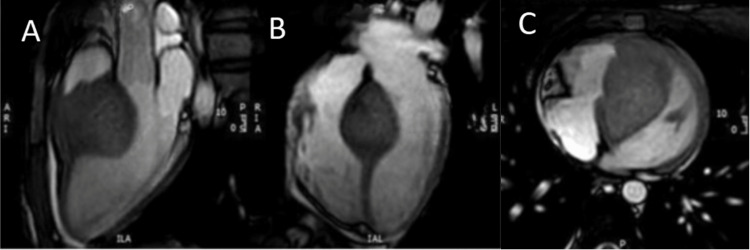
CMR imaging (A and B) SSFP of apical four-chamber and apical three-chamber images in sequence. (C) Mid axial SSFP image showing continuity with the free wall. Large interventricular mass involving the basal-mid septum measuring 5 x 3 cm. CMR, cardiac magnetic resonance; SSFP, steady-state free precession
